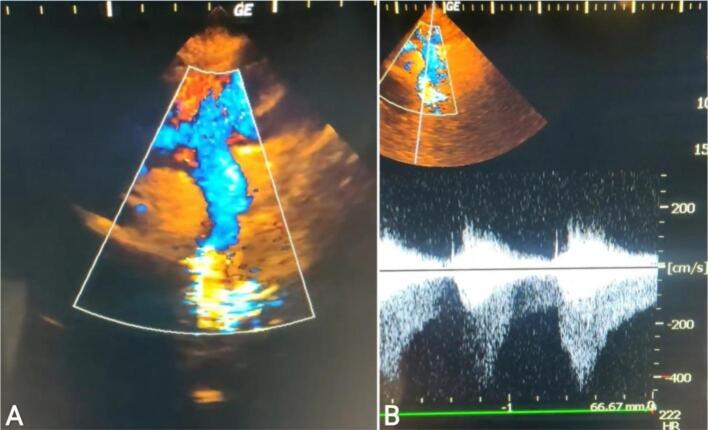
Image (A): A suprasternal view of the aorta reveals turbulent blood flow in the discending aorta, consistent with CoA. Image (B): Continuous wave doppler across the coarctation segment, presenting a peak velocity of 4 m/s and an extended speed reduction during diastole (diastolic tail).
